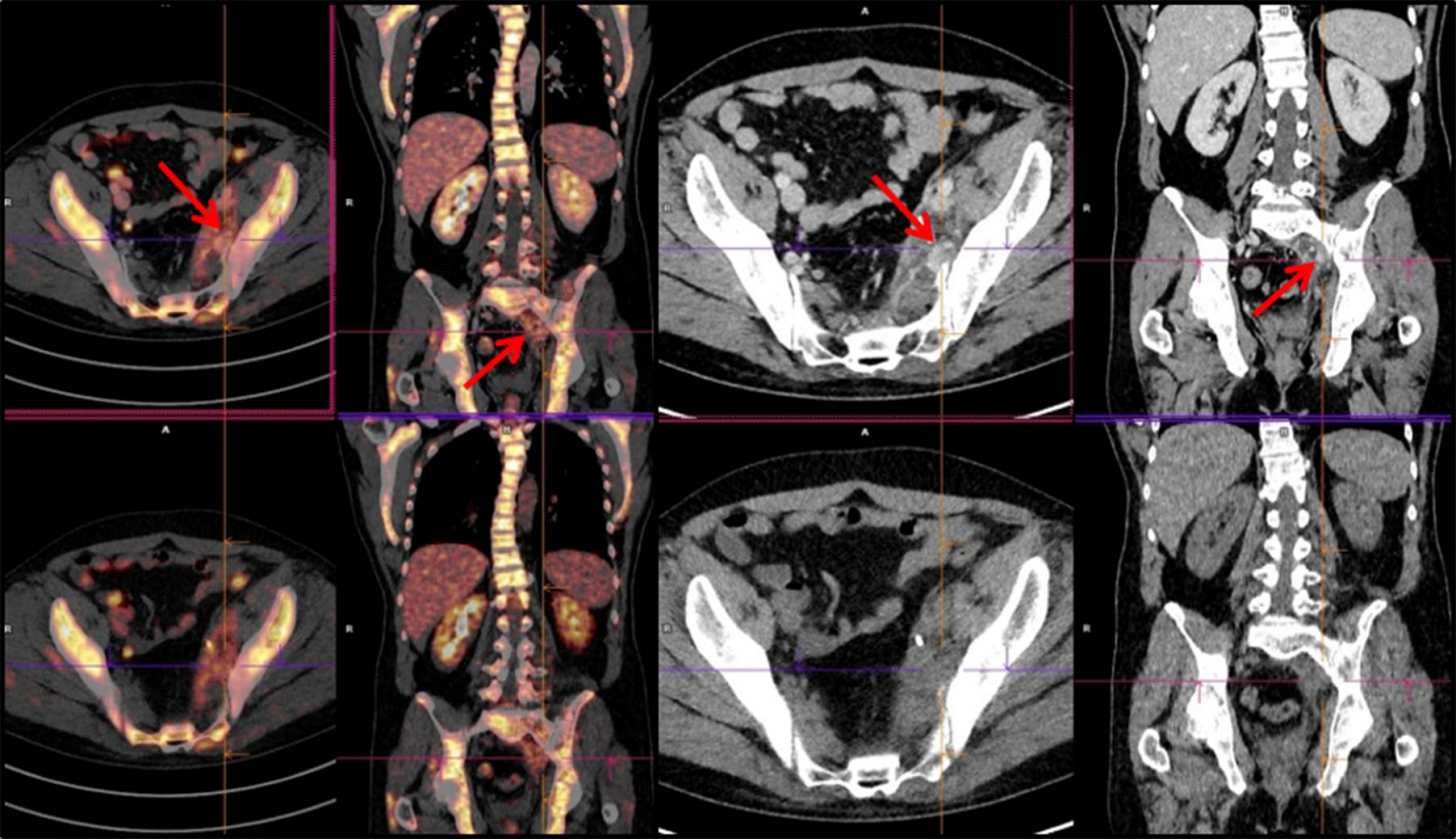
PET/ceCT showed additional nodal lesions in the left iliac region [DS 3; SUVmax 2.3].
